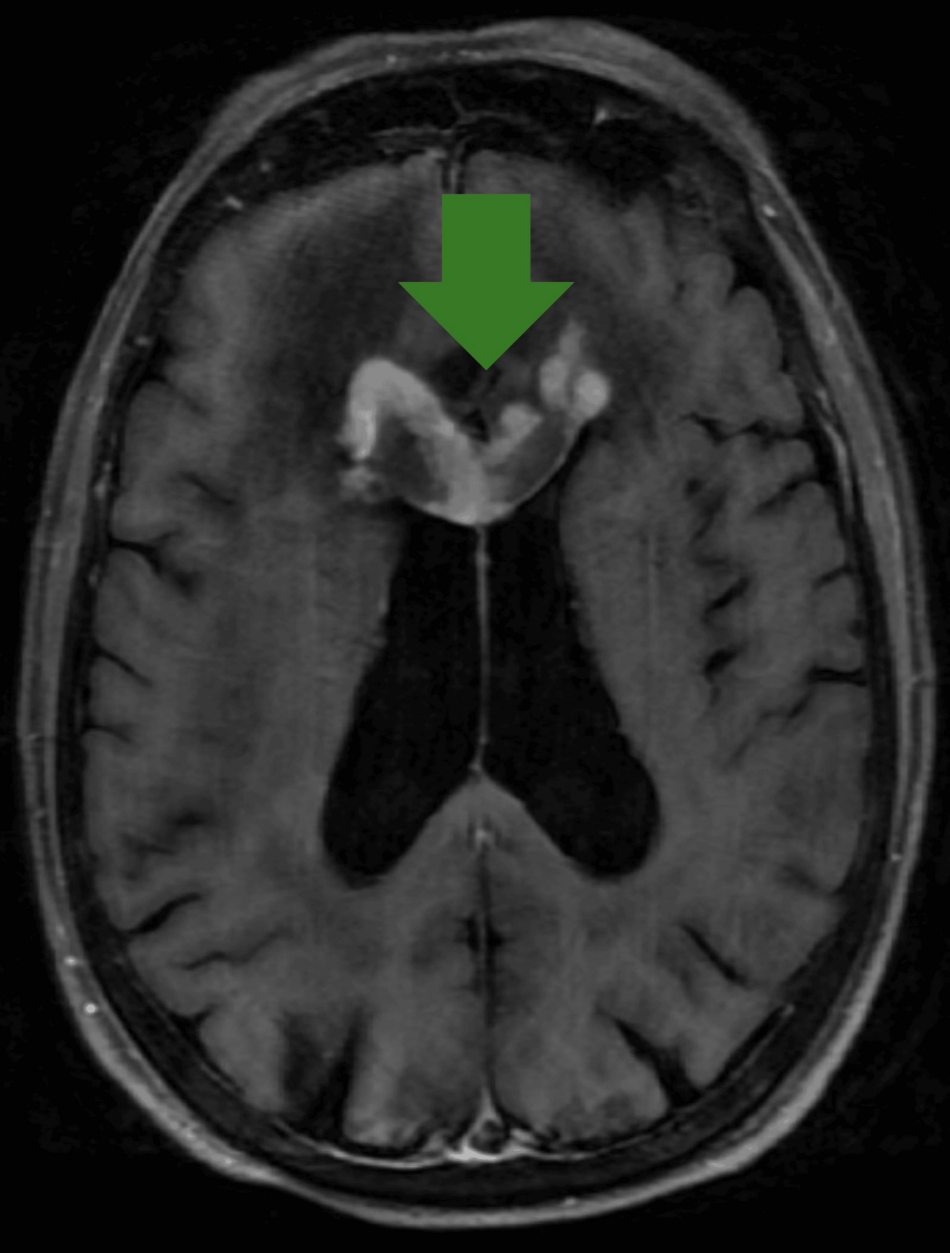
T1-weighted post-contrast MRI of the brain in patient 1 with SCNSL There is an enhancing lesion of the right genu of the corpus callosum. SCNSL = secondary central nervous system lymphoma
